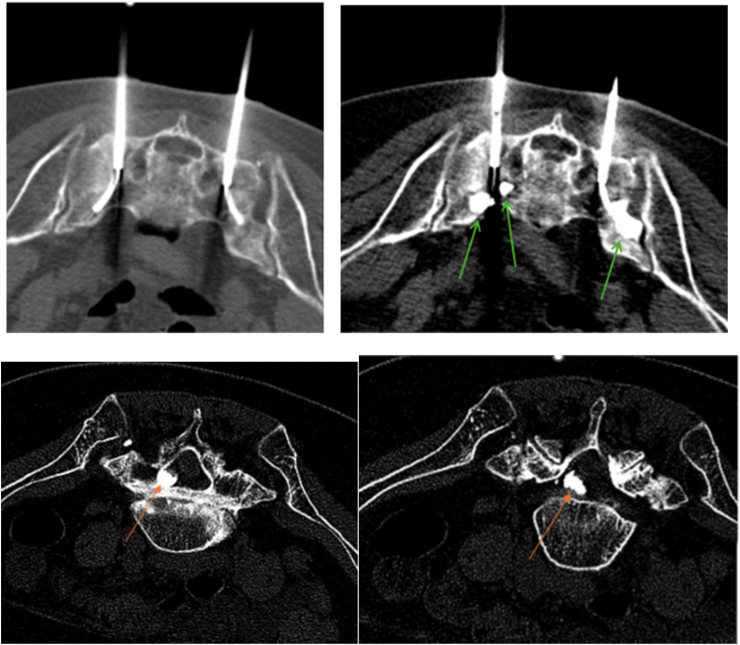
Image 1. CT-guided sacroplasty of the sacrum. The image on the top row shows CT-guided left- and right-sided needle (cannula) placement for sacroplasty prior to cement injection. The image on the right shows cement injection during sacroplasty, with the left-sided needle showing lateral flow of polymethylmethacrylate within the sacrum. Cement delivered through the right-sided needle shows both medial and lateral dispersion, with medial cement extravasation extending toward adjacent neural structures.* The second-row images are post sacroplasty CT showing cement extravasation (arrow)posterior to L5 and S1 vertebrae within the spinal canal.
